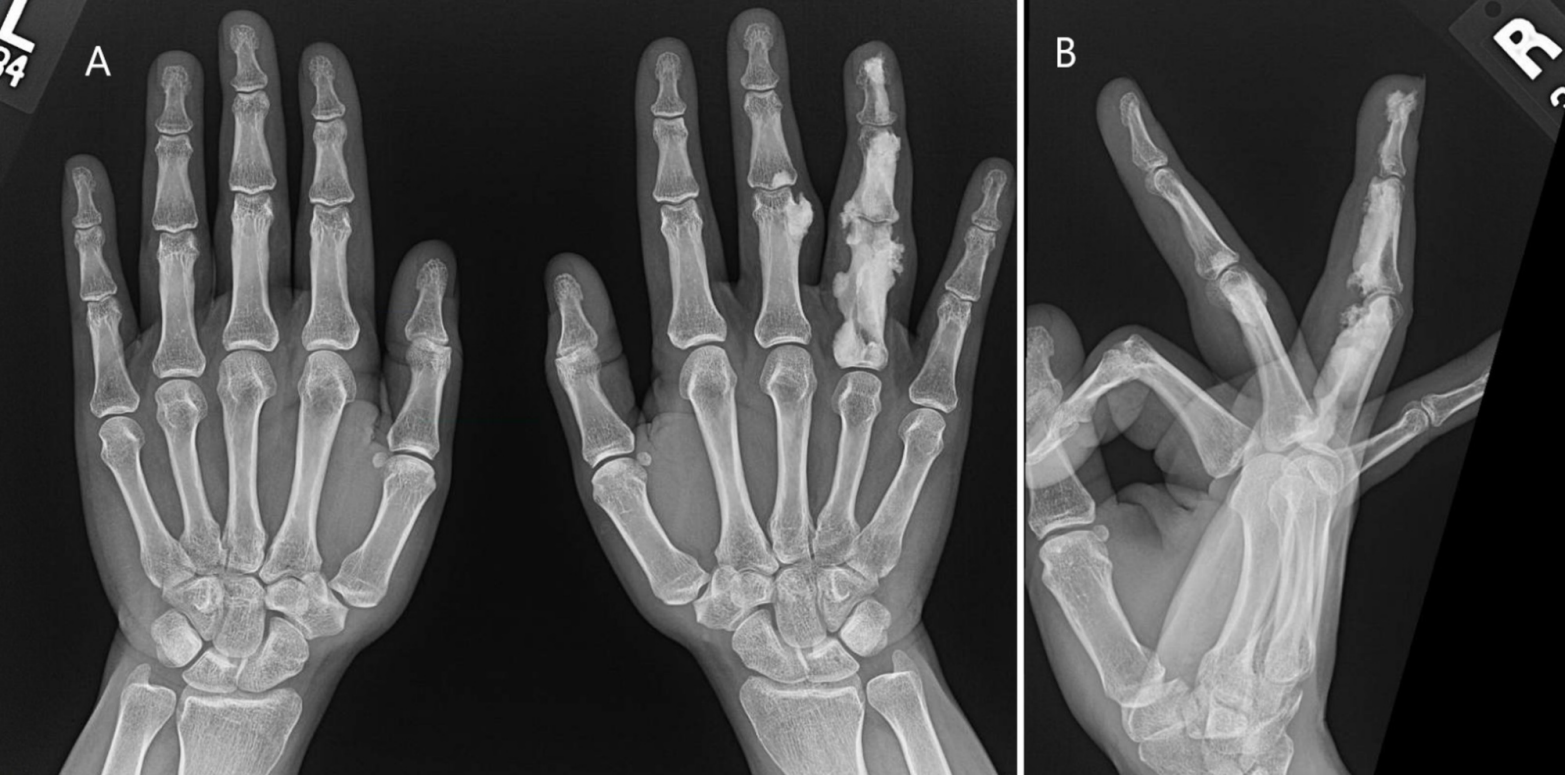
Plain X-ray: A - Bilateral hands. B - Right hand.
